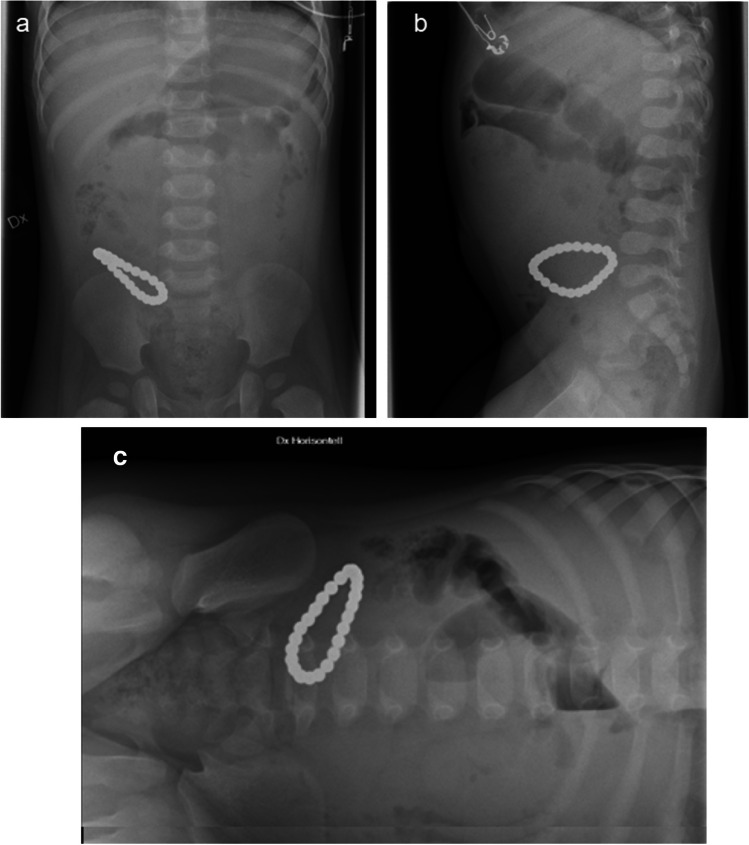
Anteroposterior (a), lateral (b) and left lateral decubitus (c) abdominal radiographs of a 1-year-old boy who presented at the emergency department with vomiting and a clinical suspicion of ileus or gastroenteritis. The radiographs reveal a radiopaque pearl bracelet in the bowel. The boy’s guardian denied known ingestion of foreign bodies
